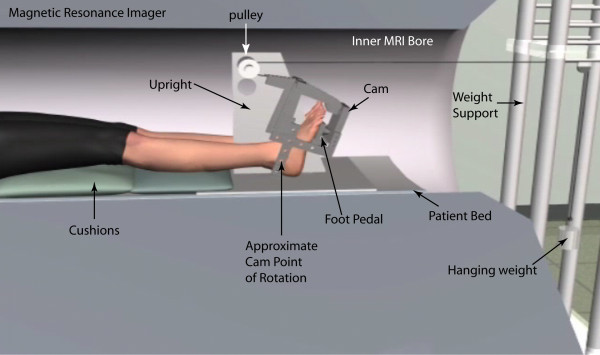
Subject placement within the MR imager.
